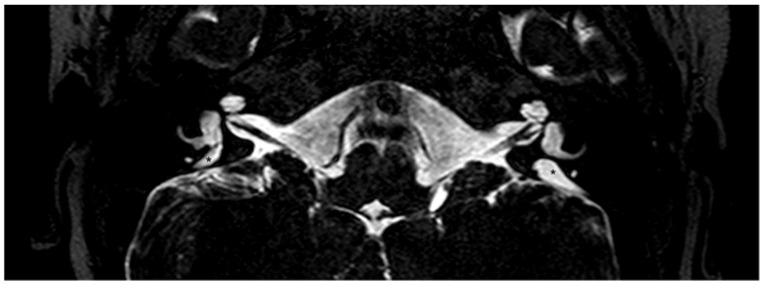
Magnetic resonance imaging of the temporal bones of the same patient shown in Figure 2, T2-weighted. A bilaterally enlarged endolymphatic duct can be observed. *, enlarged endolymphatic duct.
